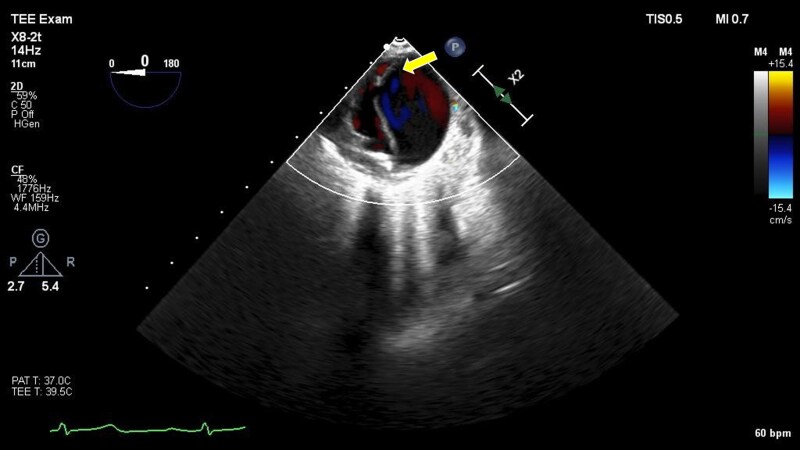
Intraoperative transoesophageal echocardiography demonstrates an intimal flap in the descending aorta (arrow).
